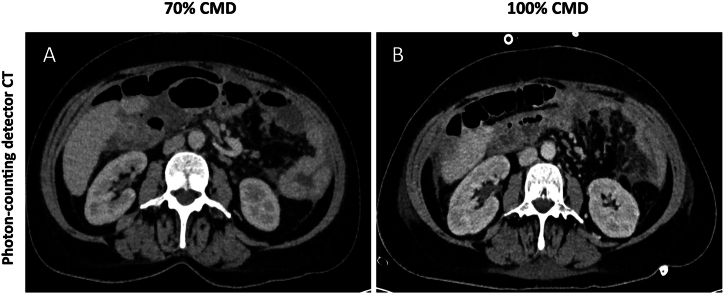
Representative axial images (window width/window level: 300/40 HU) of the same patient with 70% contrast media dose (CMD) (A) and 100% CMD (B) acquired with a photon-counting detector CT (PCD-CT).
